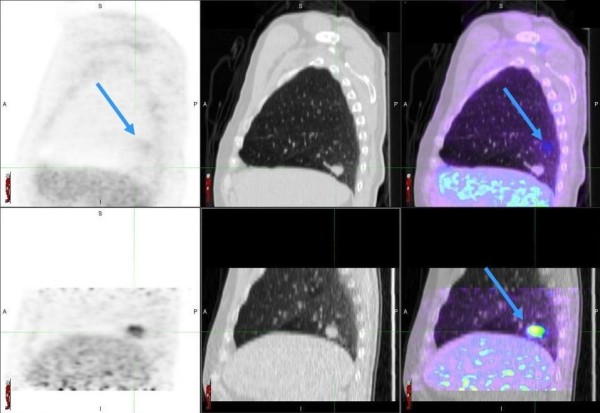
Lesion in the Right Lower Lobe was initially indeterminate based on the WB-PET/CT scan (top row - SUVmax = 1.8). The 4D-PET/CT scan (bottom row –SUVmax = 4.8) revealed FDG uptake in the lesion influencing the reporting physician to re-classify the lesion as probably malignant. The blue arrow in the top left hand cell shows how the significant mis-registration between the PET and CT scans is then well corrected on the 4D-PET/CT in the bottom right hand panel.
